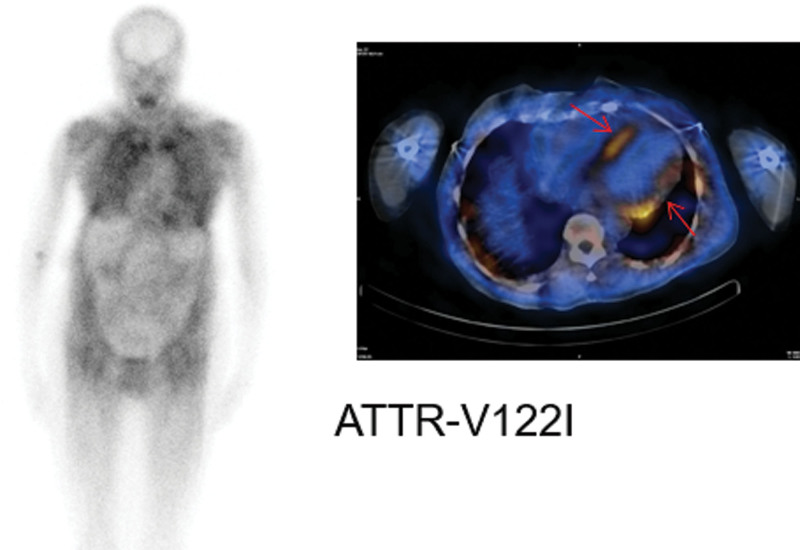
The planar whole body ^99m^Tc-DPD scan images show increased soft tissue uptake in the lungs, muscles of shoulder, pelvic girdle and in the muscles of abdominal wall with poor definition of bones. The cardiac uptake may be present but without the help of SPECT-CT, it would not be possible to be confident about uptake and distribution of cardiac uptake. This patient was diagnosed with ATTR cardiac amyloidosis after biochemical investigations.
